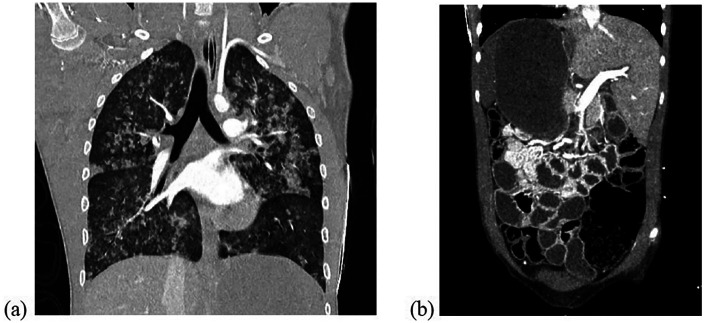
CT chest with bilateral infiltration (a) and bowel with signs of hypoperfusion after shock (b) upon admission.
